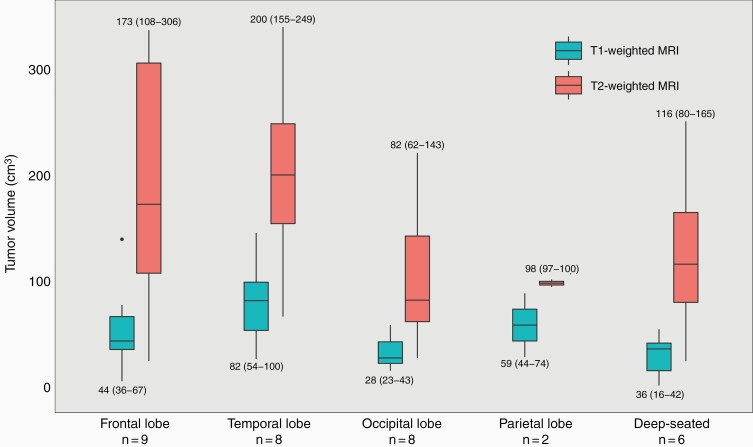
Median [interquartile range (IQR)] contrast-enhancing tumor volume in T1-weighted magnetic resonance imaging (MRI) and median (IQR) volume of tumor-associated non-enhancing hyperintense lesions in T2-weighted and/or fluid-attenuated inversion recovery (FLAIR) MRI according to tumor location in 33 patients diagnosed with butterfly glioblastoma between 01/01/2007 and 31/12/2014.
